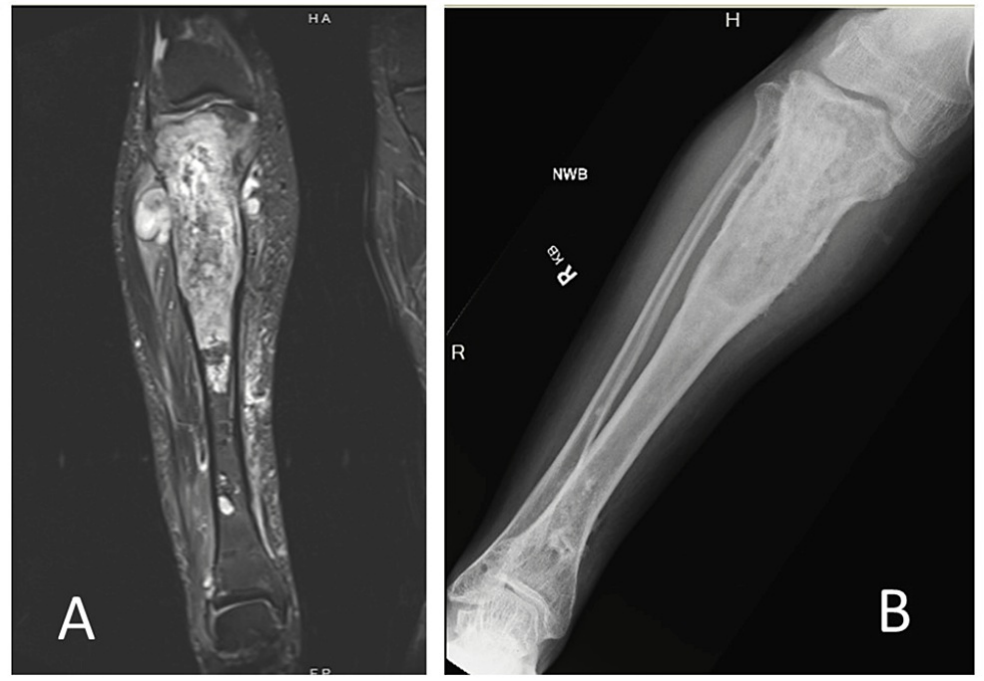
MRI (A) and radiograph (B) of the tibia. MRI: magnetic resonance imaging.
